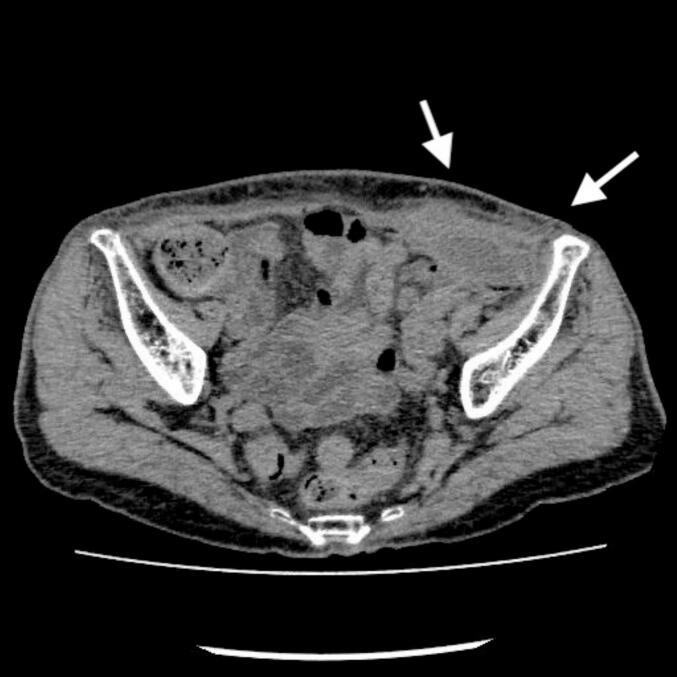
Abdominal/pelvic computed tomography image obtained on postoperative day 62 following mesh implantation for inguinal hernia repair. A massive abscess measuring 15.2 cm × 9.7 cm is observed in the Douglas pouch.
